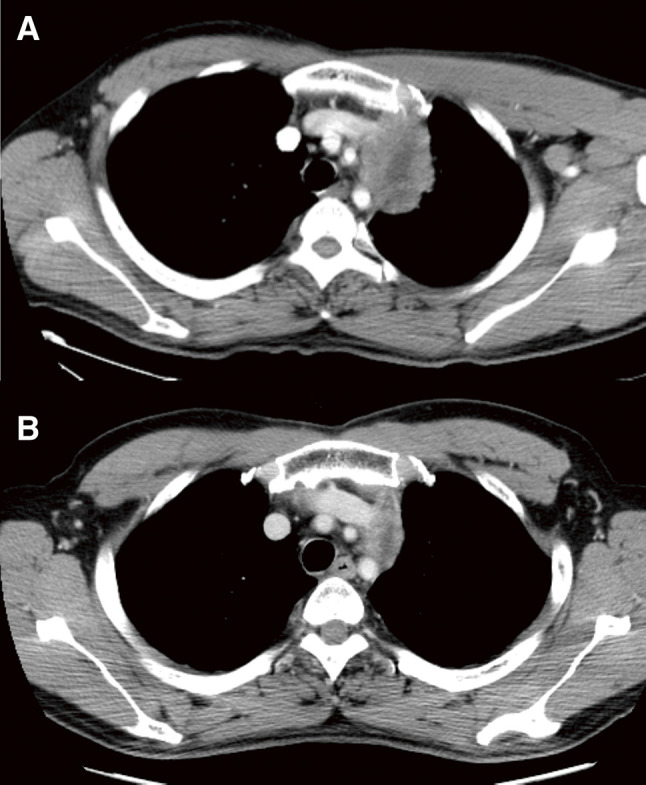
Computed tomography showed an anterior mediastinal tumor. The size was (A) 7.0 × 5.5 cm^2^ after diagnosis and (B) 3.8 × 2.0 cm^2^ after chemoradiotherapy. Tumor invasion to the left lung and left innominate vein was suspected. LIV, left innominate vein
